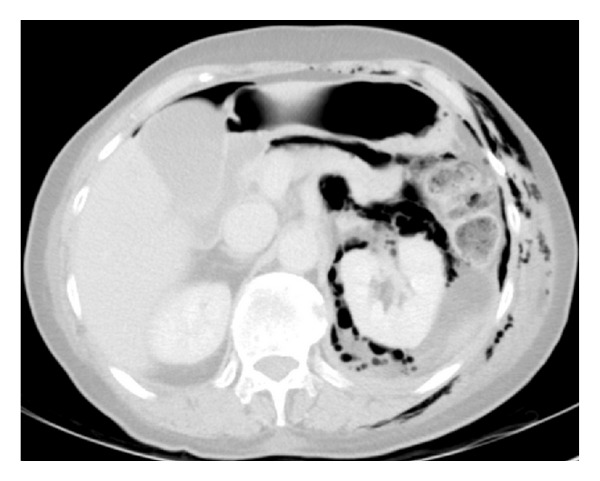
Abdominal axial computed tomography (CT) showing intraperitoneal free air following unrecognized bowel perforation.
